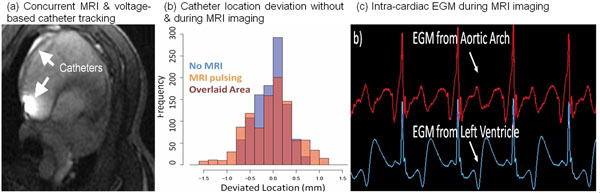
a) MRI shows catheters in the coronory sinus and the right atrium b) frequencies of deviated location of catheter electrode with no MRI (blue), with MRI pulsing (orange), and their statistically overlaid area (red). c) clean intra-cardiac EGM traces measured from catheters during MR imaging.
